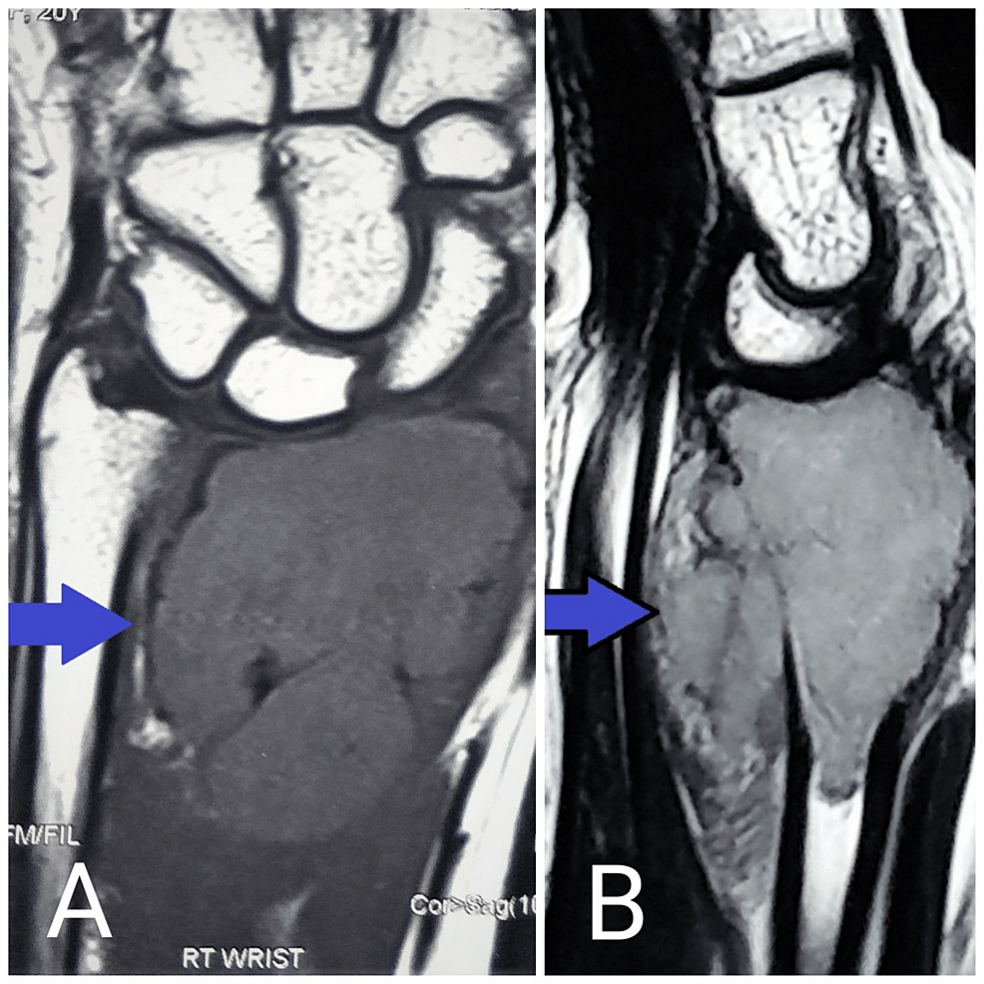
MRI of wrist showing cortical breech, medial and volar soft tissue involvement by tumor
